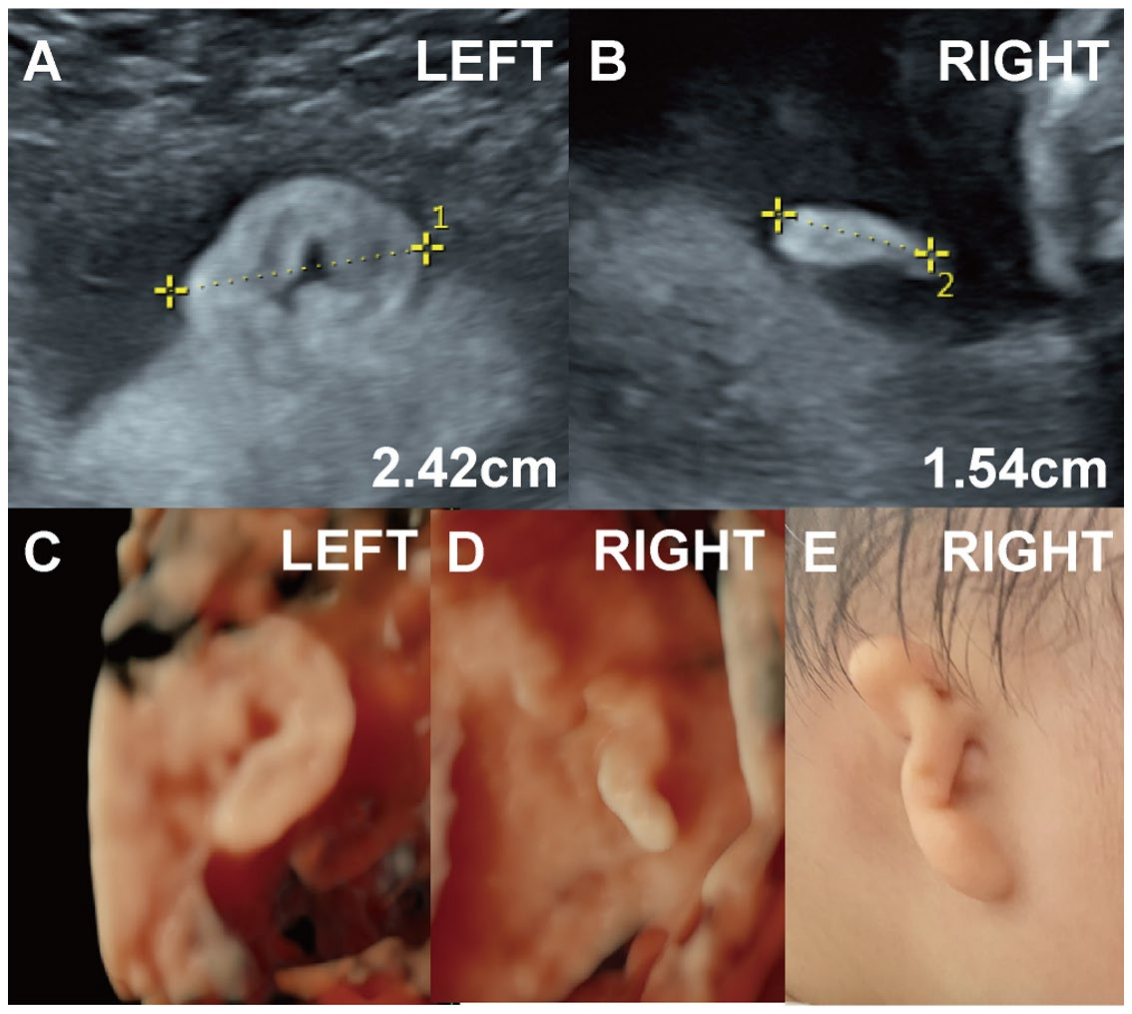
(Case 6). (A,B) A systemic ultrasound at 22 weeks revealed an abnormal right ear. Two-dimensional imaging showed a right ear length of approximately 1.5 cm with a slightly elevated, ribbon-like, peanut-shaped helix. Genetic testing was normal. (C,D) Three-dimensional ultrasound was also performed. (E) The pregnancy resulted in the full-term delivery of a baby girl with right-sided Grade II microtia and external auditory canal atresia. Hearing tests at 11 months confirmed conductive hearing loss in the right ear.
